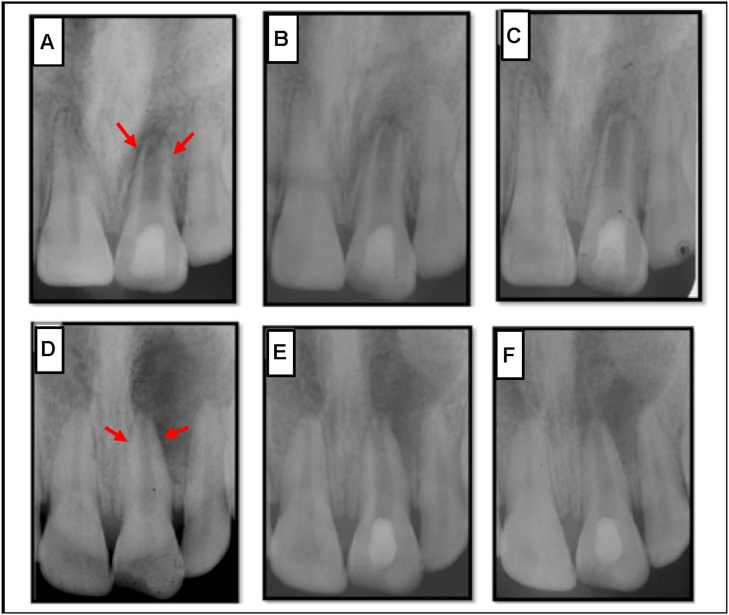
X-ray of teeth with revascularization. A-C) Show teeth with revascularization. A) The dentinal walls (red arrows) for this patient, a 9 year old girl, are thin with a larger opening at the apex. B) after 6 months, there is calcification present at the apex. C) After 1 year, revascularization of the tooth is achieved, primarily through the bridge composed of calcium at the apical section and root lengthening. D-F) Show teeth with revascularization and PRP. D) Similar to above, the dentinal walls (red arrows) are thin with a larger opening at the apex for this patient, a 15 year old boy. This patient underwent a treatment an identical procedure however supplemented with PRP. E) At 6 months, the calcium barrier was reduced at the apex compared to the 9 year old girl and the patient reported to be symptom free. F) After 1 year, revascularization of the tooth is successful and is comparable to a normal apical tooth. Reprodcued with permission [44]. 2021, J. Endo.
